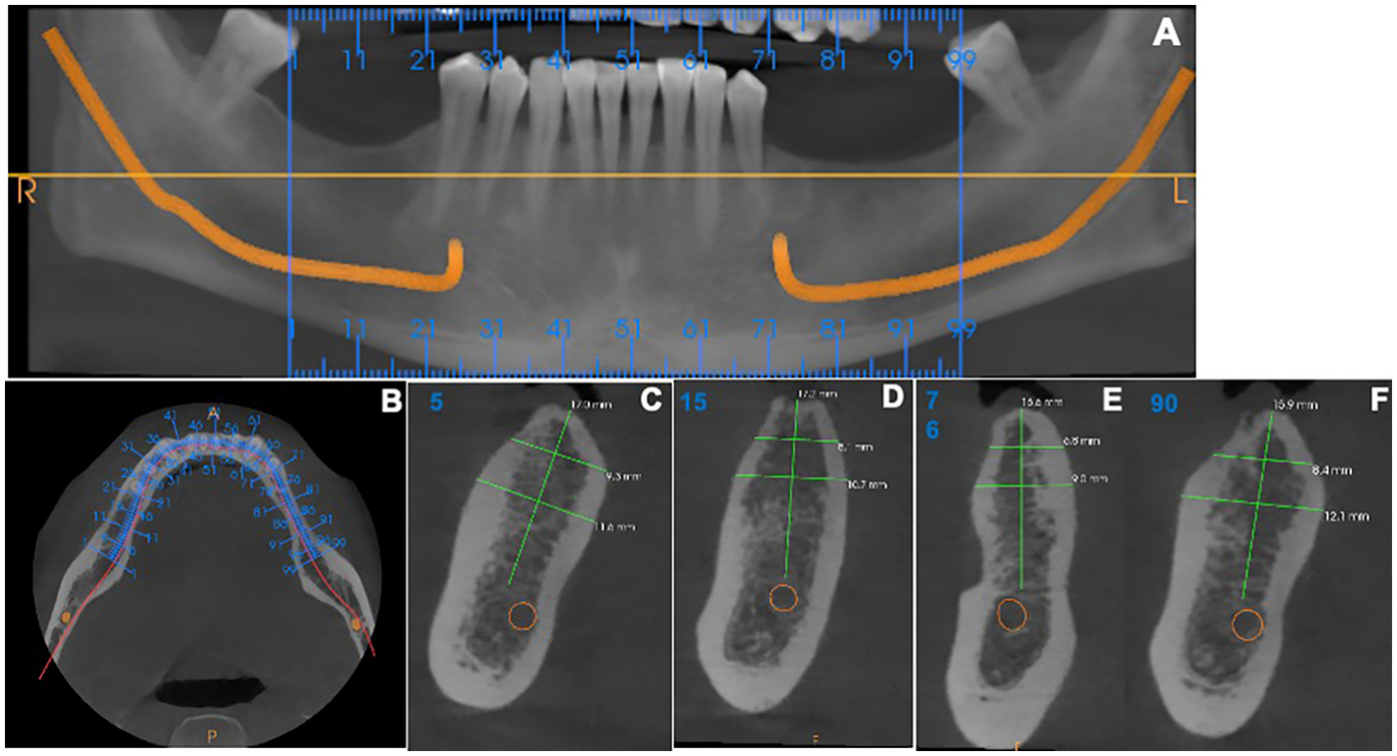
Cone-beam computed tomography images for patients included in the study. (A) An overview of the alveolar bone level by orthopantomography. (B) Axial view for the implant sites. (C–F) Sagittal view for measuring the alveolar bone level.
